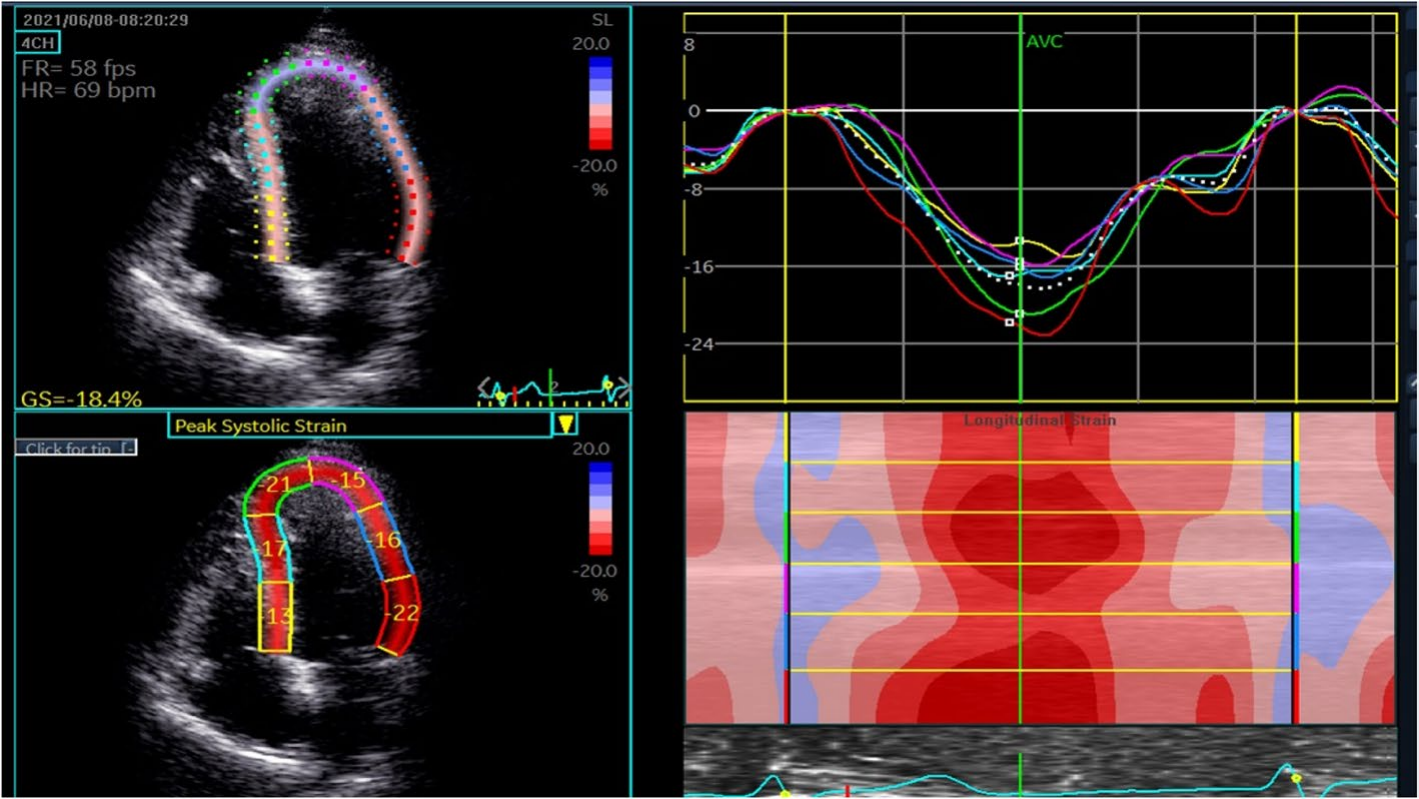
Acquisition of left ventricular apical four-chamber strain imaging by two-dimensional speckle tracking echocardiography in type 2 diabetic patients
